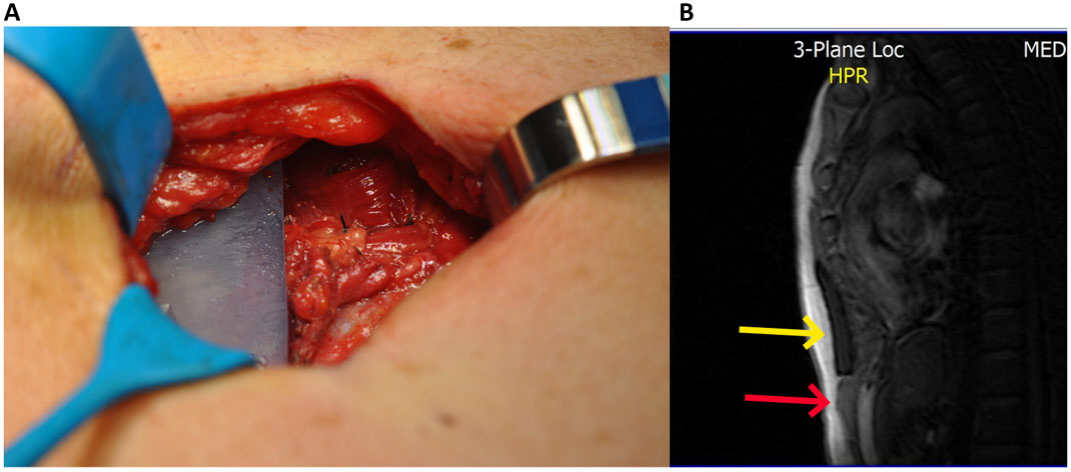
Intraoperative view. (A) The inferior edge of the implant is in direct contact with the superior sutured edges of the rectus abdominis muscles. (B) Magnetic resonance image: the superior arrow indicates the silicone implant and the inferior arrow indicates the rectus abdominis muscles.
